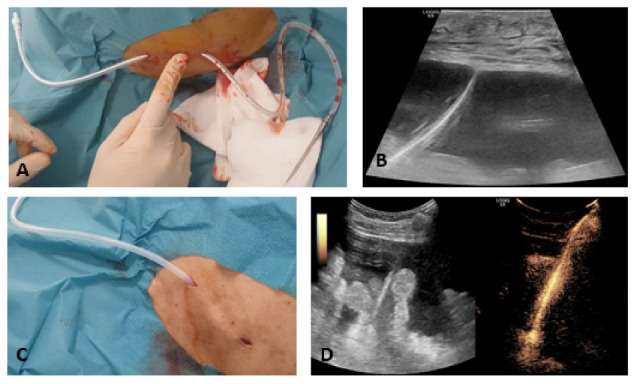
(A–D): Subcutaneous tunnelling of the PleurX catheter. The fingertip points to the position of the polyester cuff (A); sonographic control of guidewire position in the abdominal cavity (B); after using the peel-away introducer, the fenestrated part of the catheter is now inside the abdominal cavity (C). Optionally, the position of the catheter in the abdominal cavity can be verified by administering a few drops of SonoVue over the drainage (D).
